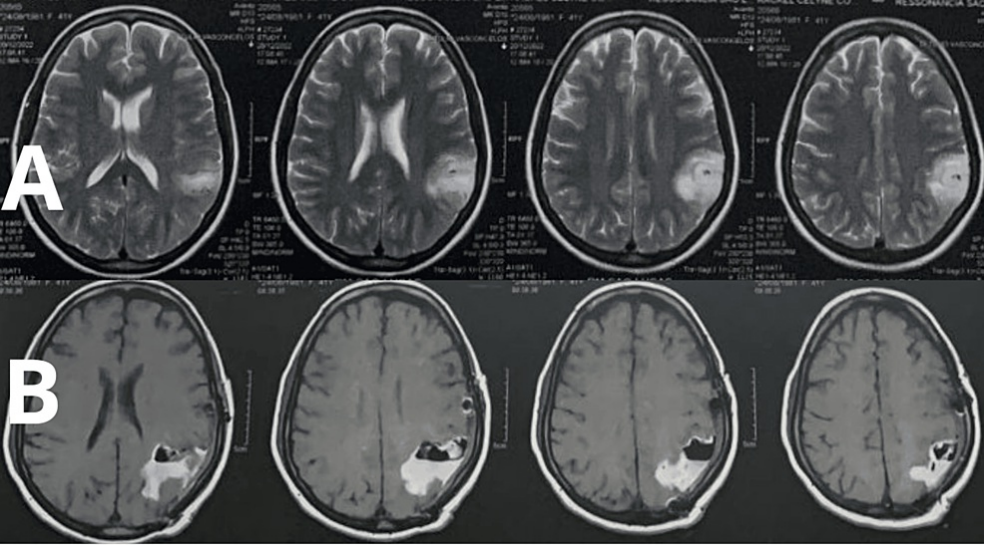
Original image demonstrating magnetic resonance imaging. (A) Before the procedure. (B) One week after the procedure.
